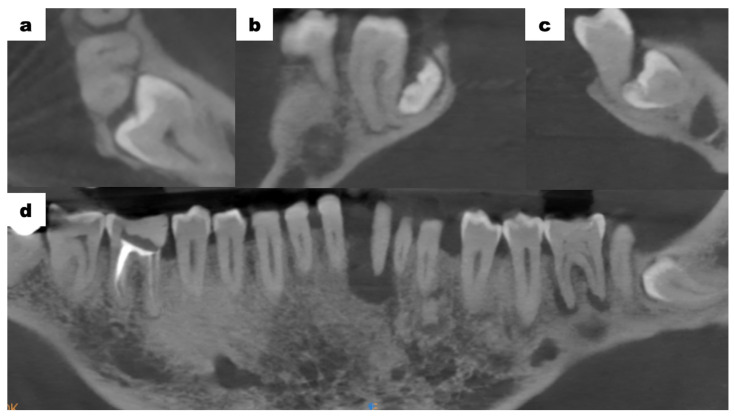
Mandibular CBCT examination scans before initiation of treatment; (a) an axial plane scan—tooth 38 retained in the horizontal, mesial-angular position; (b) a sagittal plane scan—tooth 37 distally exposed due to the adjacent position of tooth 38, no signs of root resorption; (c) a frontal plane scan—between tooth 37 and 38, visible potential plaque retention; (d) anteroposterior projection—bone thinning in the chin region.
